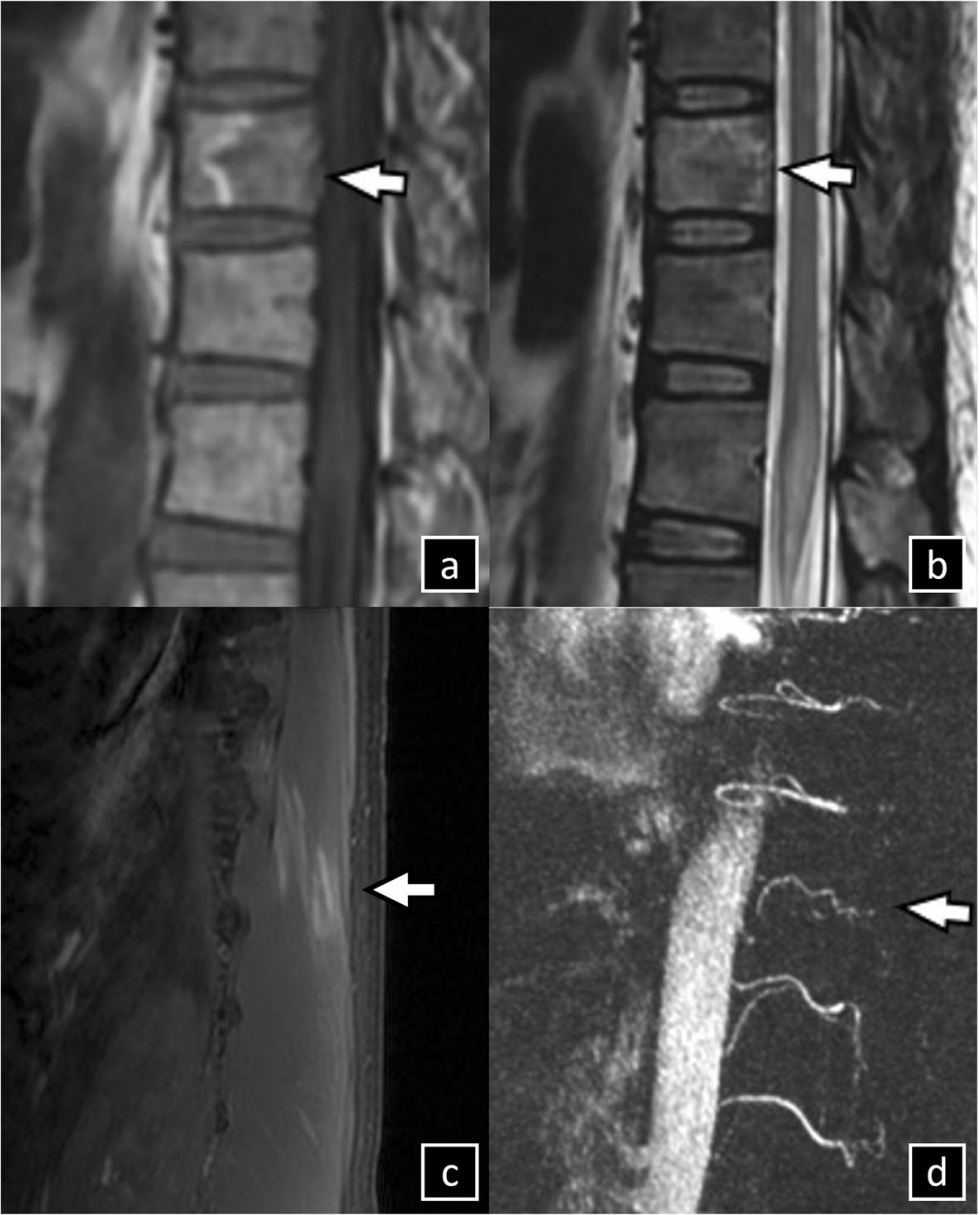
Sagittal view of spine MRI of patient 1. a High signal intensity on T1-weighted image and (b) low signal intensity on T2-weighted image at vertebral body T9 indicate vertebral bone infarction. c High signal intensity on apparent diffusion-coefficient image in erector spinae and (d) decreased blood flow in the supply artery on time-of-flight MRI at T10 also indicate muscle infarction. MRI, magnetic resonance imaging
